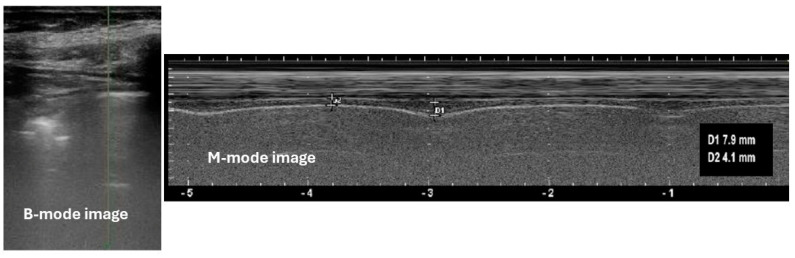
Ultrasonographic appearance of diaphragm thickness in an older subject with heart failure during quiet breathing. The diaphragm is visualized in the B-mode image as the track-like structure lying between two hyperechoic parallel lines. Measures of thickness during inspiration and expiration on quiet breathing are taken after activating the M-mode ultrasound function. The distance between the two parallel hyperechoic lines appears to increase in synchrony with inspiration (measure of thickness 7.9 mm) and to decrease during expiration (measure of thickness 4.1 mm).
